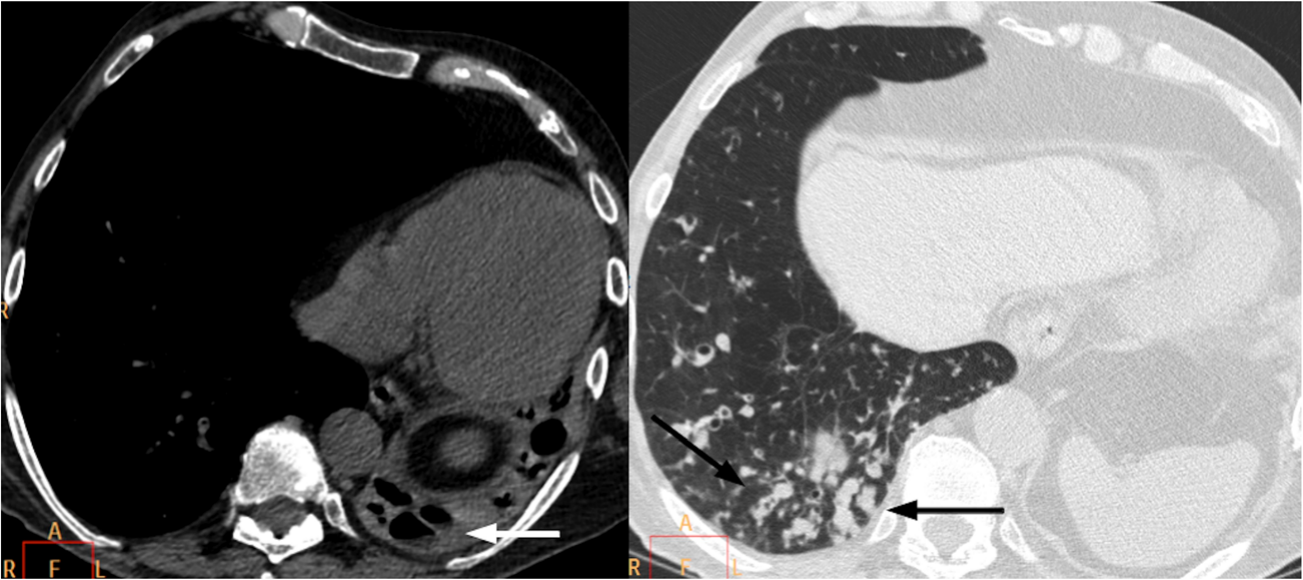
A 73-year-old male with a history of pulmonary TB as a youth and chronic obstructive pulmonary disease was seen for fever and purulent expectoration. Unenhanced multislice CT depicted complete left pulmonary destruction with residual cystic bronchiectasis associated with air-fluid levels (white arrow in the right image) and airway occupation with mucus impaction in the lower right lobe (black arrows in the left image). Sputum culture was positive for *Pseudomona aeruginosa* and *Streptococcus pneumoniae*. Antibiotic treatment was established without success, and the patient died 15 days after hospitalisation
